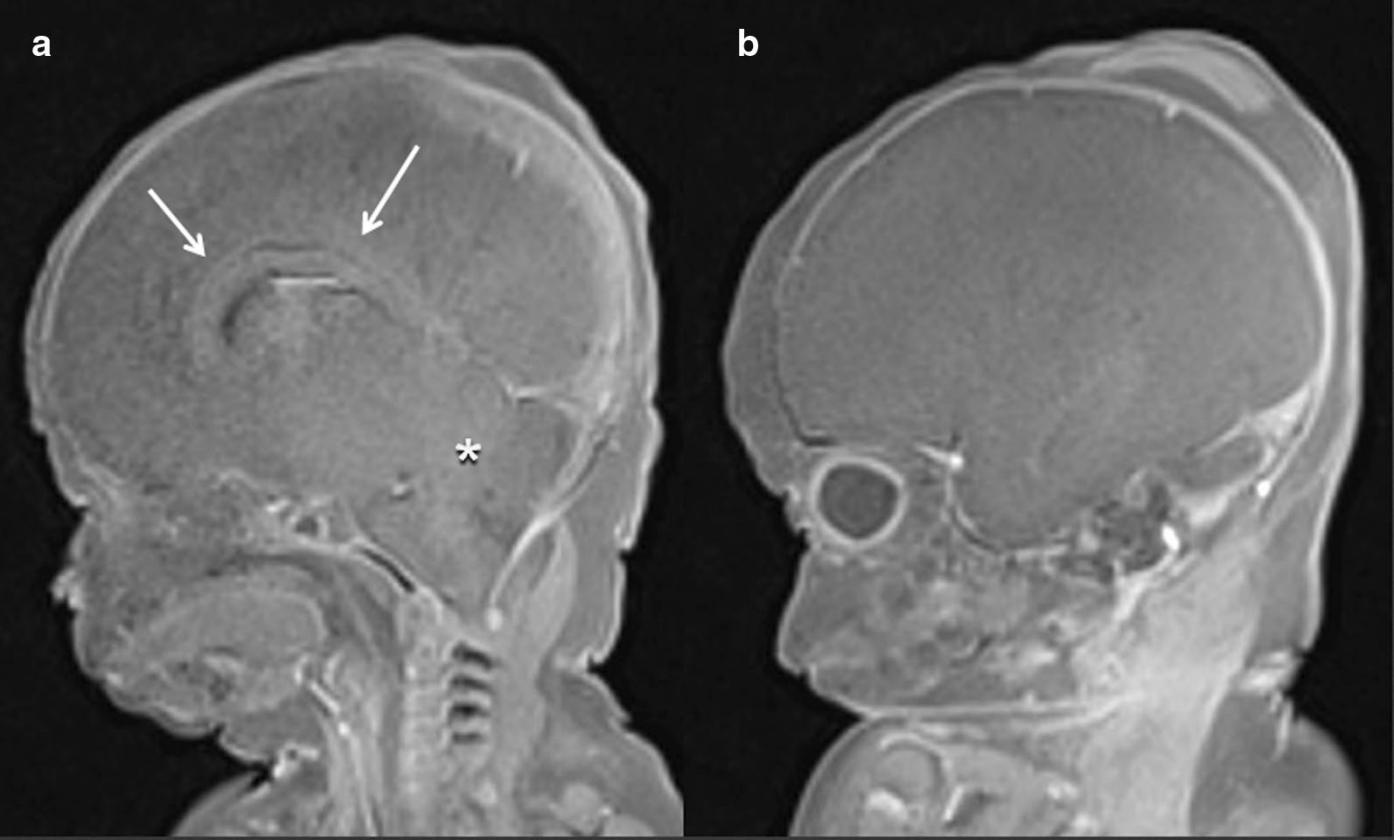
25-week foetus with normal cerebral lamination. a, b Sagittal T1-weighted images, normal midline corpus callosum (arrows) and posterior fossa (asterisk)
